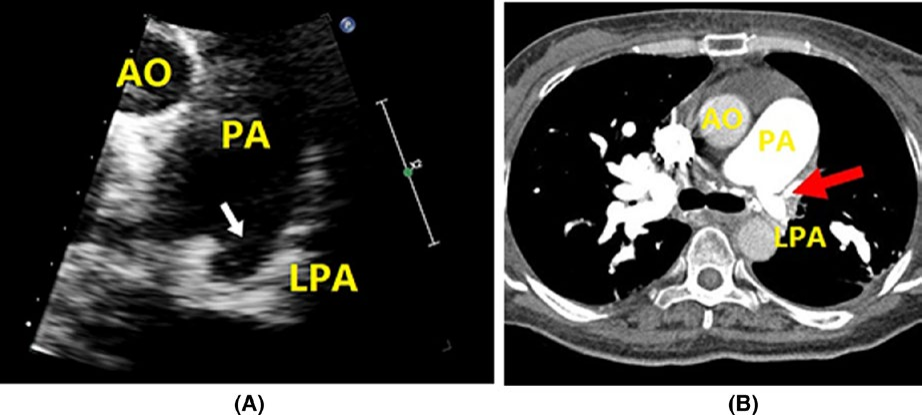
A. Parasternal aortic short‐axis view showed short left pulmonary artery and blind end (arrowheads) (movie clip S9). B. CTPA showed short left pulmonary artery and occluded left pulmonary inferior artery (arrow heads). AO = aorta; PA = pulmonary artery; LPA = left pulmonary artery
